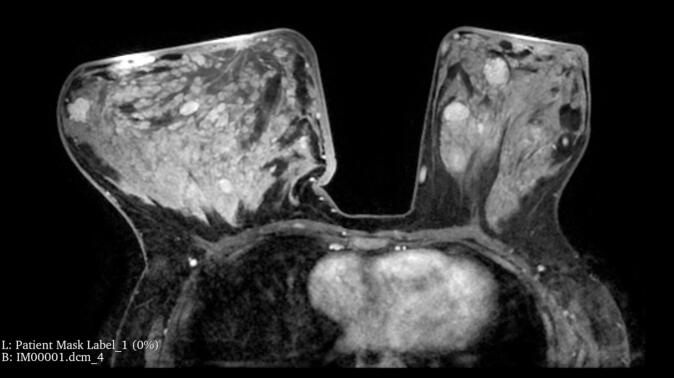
Preoperative radiologic findings: magnetic resonance imaging findings. Severely dense fibroglandular tissue, diffuse parenchymal inflammation with skin thickening, and areolar edema in the right breast.
